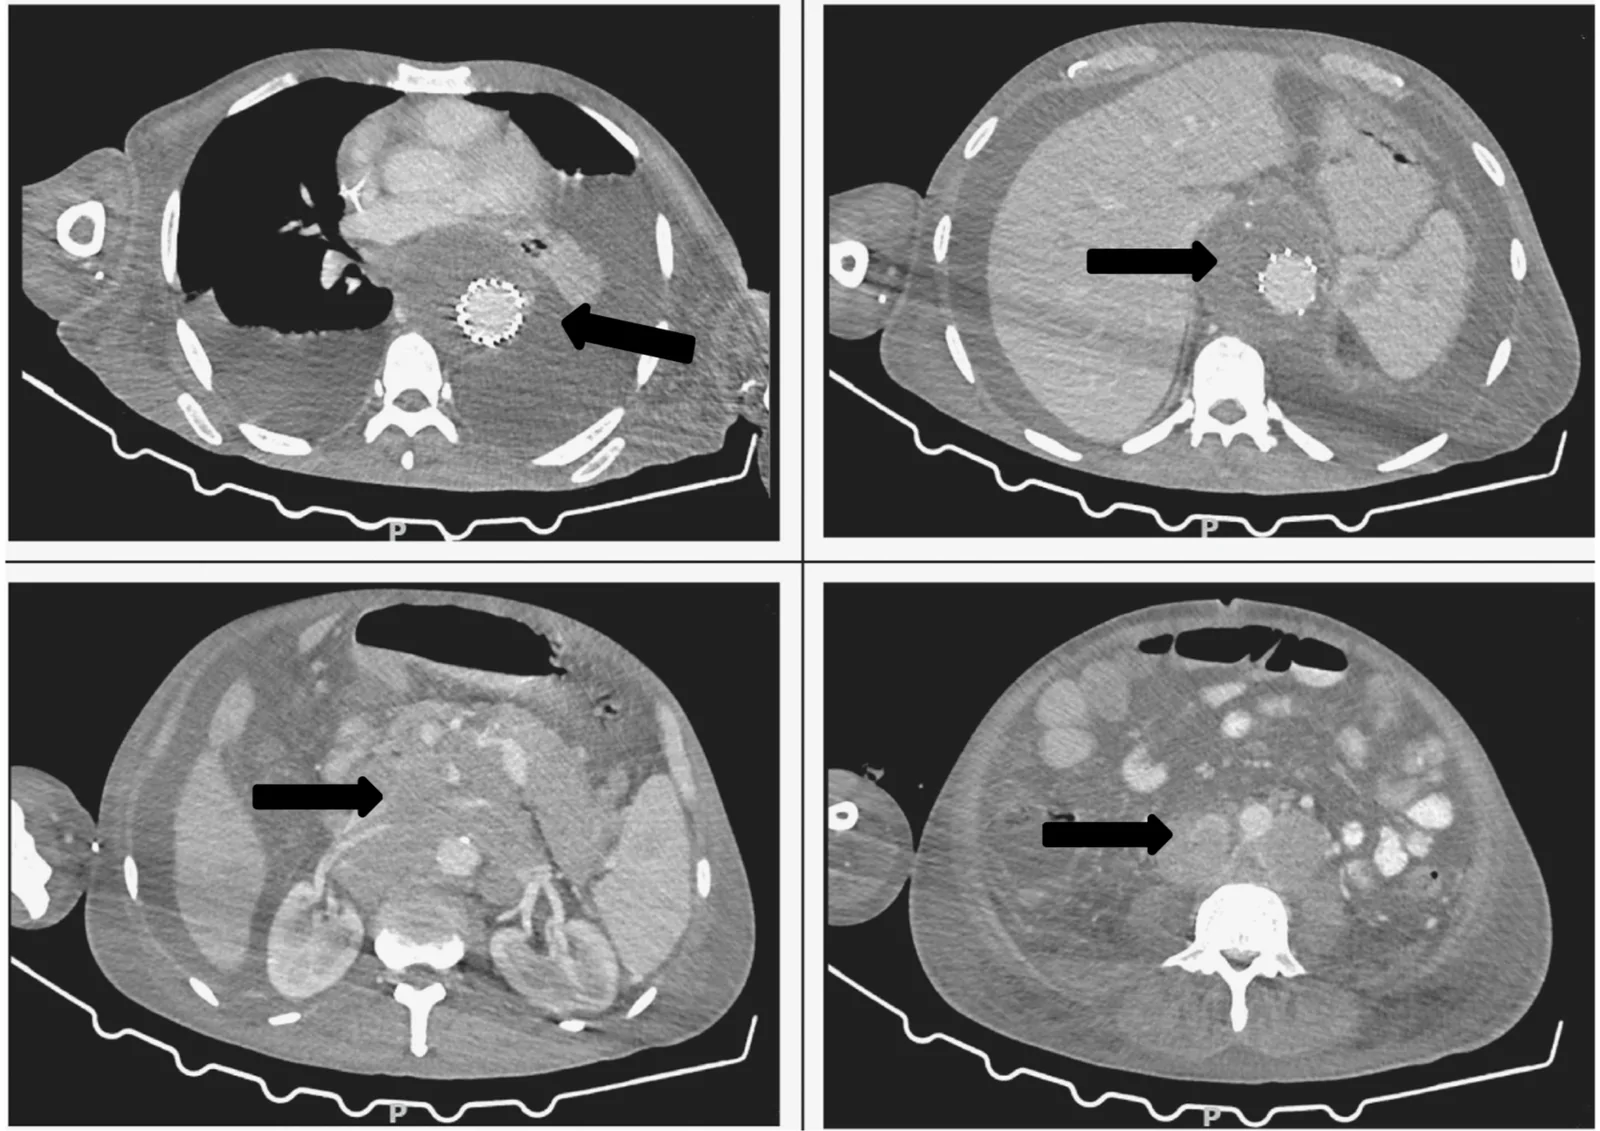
Contrast-enhanced abdominal computed tomography (CT). Axial views demonstrating a bulky retroperitoneal lymph node conglomerate encasing major abdominal vessels, alongside focal splenic and pancreatic infiltration. These findings correspond to the advanced underlying classical Hodgkin lymphoma driving the paraneoplastic immune response.
